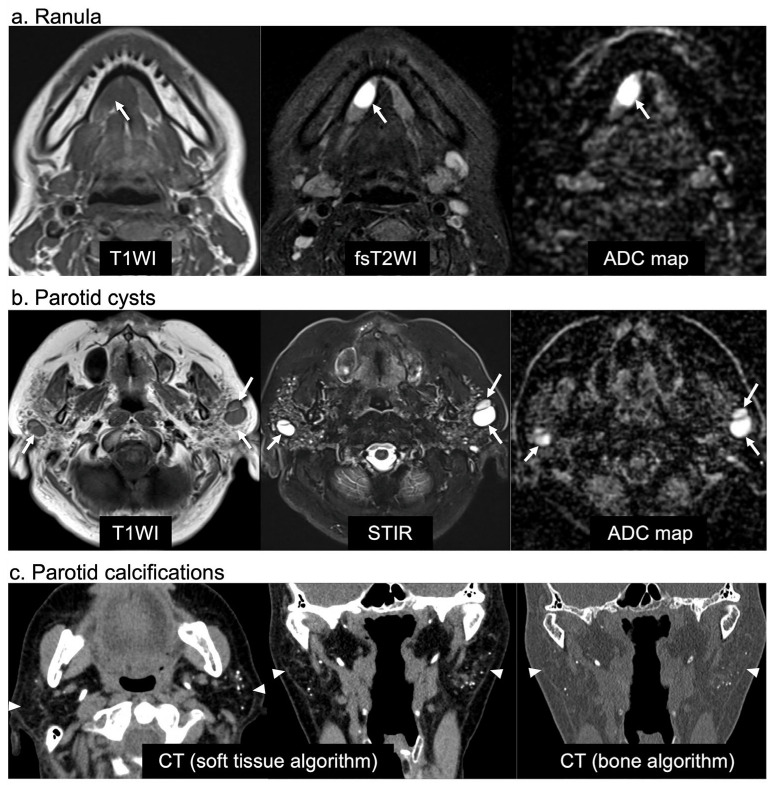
MR and CT images of SS patients. (a) Axial MR images of a 32-year-old woman with a right simple ranula (arrows). The ranula is a marked and homogeneous hyperintense area on fsT2WI with a high ADC (2.3 × 10^−3^ mm^2^/s). (b) Axial MR images of a 62-year-old woman with multiple bilateral parotid cysts (arrows) and a high ADC (2.0–2.1 × 10^−3^ mm^2^/s). The bilateral parotid glands also exhibited heterogeneous fatty areas on the T1WI and multiple hyperintense spots on the STIR image. (c) Axial and coronal CT images of a 68-year-old woman with multiple punctate calcifications in the bilateral parotid parenchyma (arrows). The bilateral parotid glands exhibit marked fatty degeneration. T1WI, T1-weighted image; fsT2WI, fat-suppressed T2-weighted image; ADC, apparent diffusion coefficient; STIR, short tau inversion recovery.
